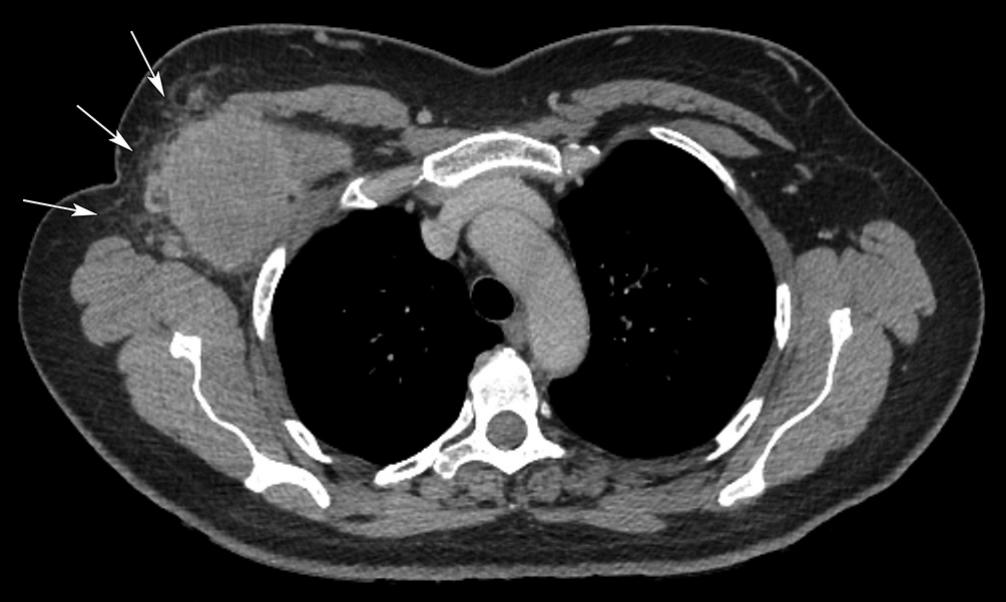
Axial post contrast CT of the axillae, confirms a poorly defined soft tissue mass in the right axilla. Note distortion and stranding of the overlying subcutaneous fat (arrows) and infiltration of chest wall/pectoralis minor musculature.
